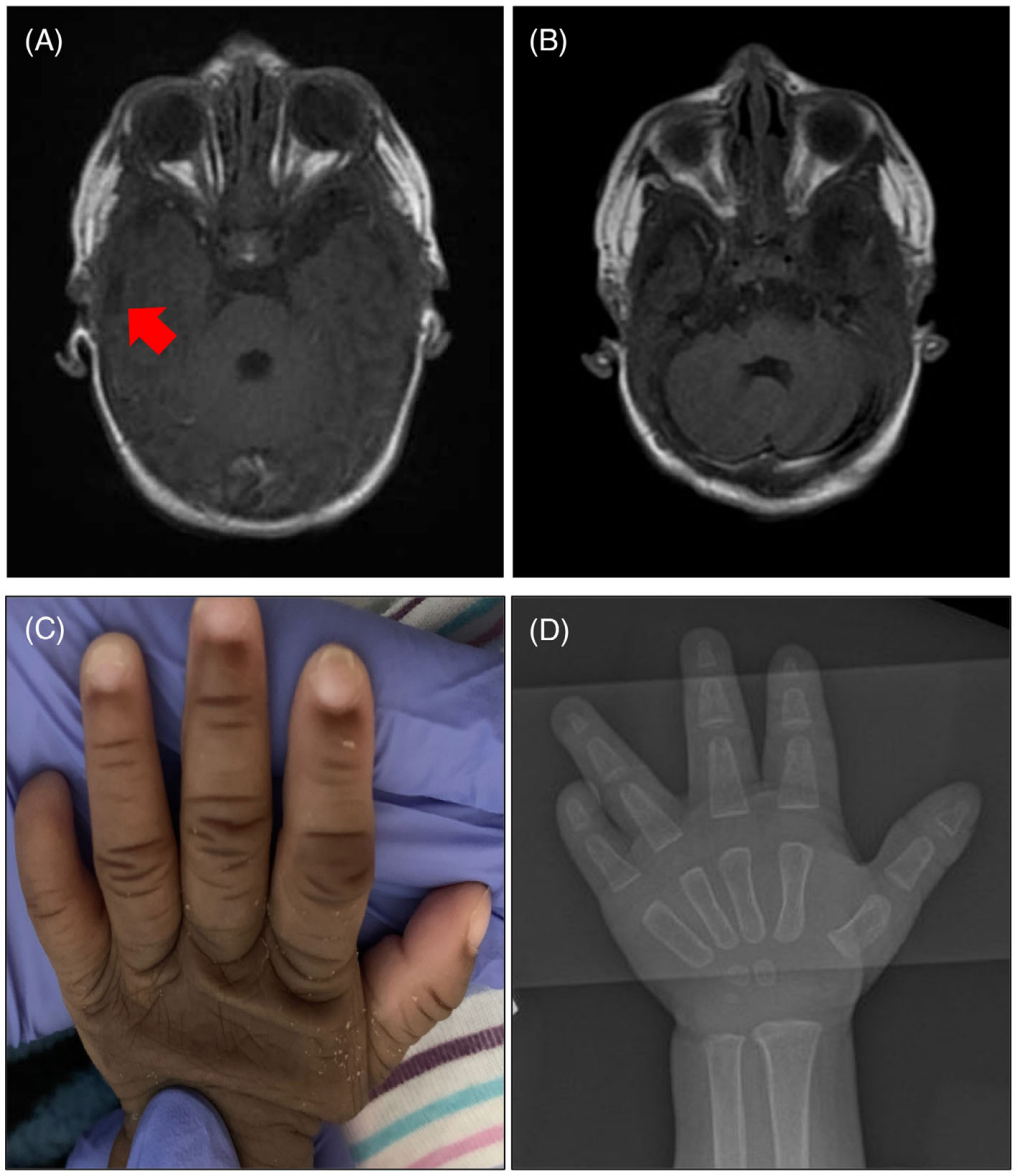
Brain MRI T1 sequences (obtained at 1 month of age). (A) Left temporal cyst. (B) Enlargement of the fourth ventricle. (C) Upper view of left hand showing wrinkled skin, nail dystrophy of digits 1–4, and nail aplasia of digit 5. This feature was present bilaterally. (D) Radiograph of left hand showing an absent distal phalanx of the 5th finger. This feature was present bilaterally.
